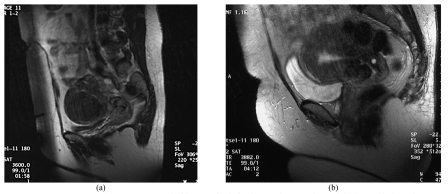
A 50-year-old woman presented with menorrhagia for the past three months. An initial MRI (a) showed the presence of an anterior intramural fibroid. She underwent a UAE and a follow-up MRI at 1 year (b) showed complete absence of the fibroid.
